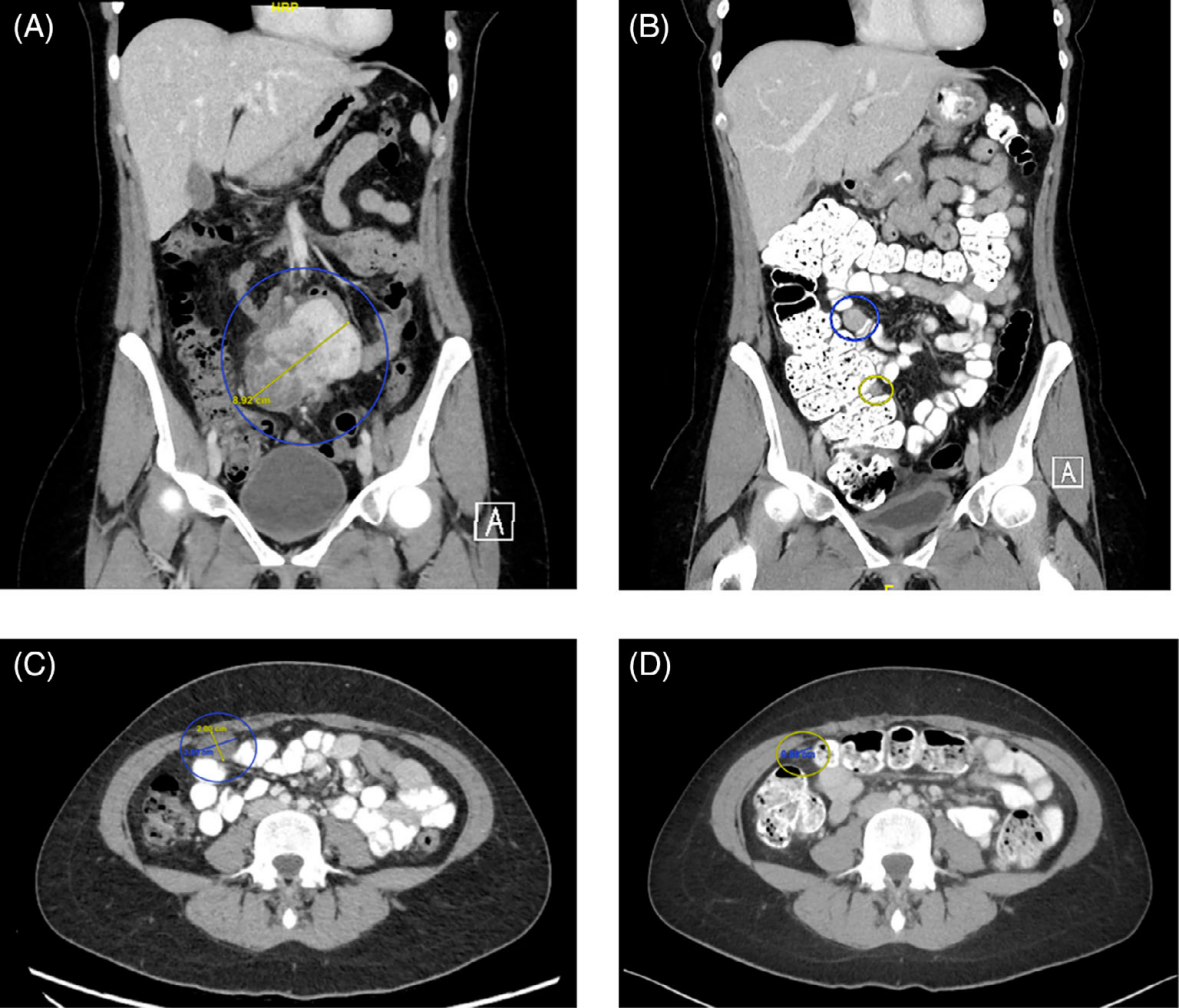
Computed tomography of the abdomen and pelvis. (A) Coronal images at time of diagnosis, demonstrating an 8.9 cm mesenteric mass. (B) Coronal images obtained 4 months after resection, with circles indicating sites of disease recurrence. (C) Axial image obtained 3 months after initiation of alectinib treatment. A 2 cm tumor mass is circled. (D) Axial image at similar level as that in image (C), obtained 14 months after initiation of alectinib treatment. The previously noted mass is now less than 1 cm maximal dimension.
